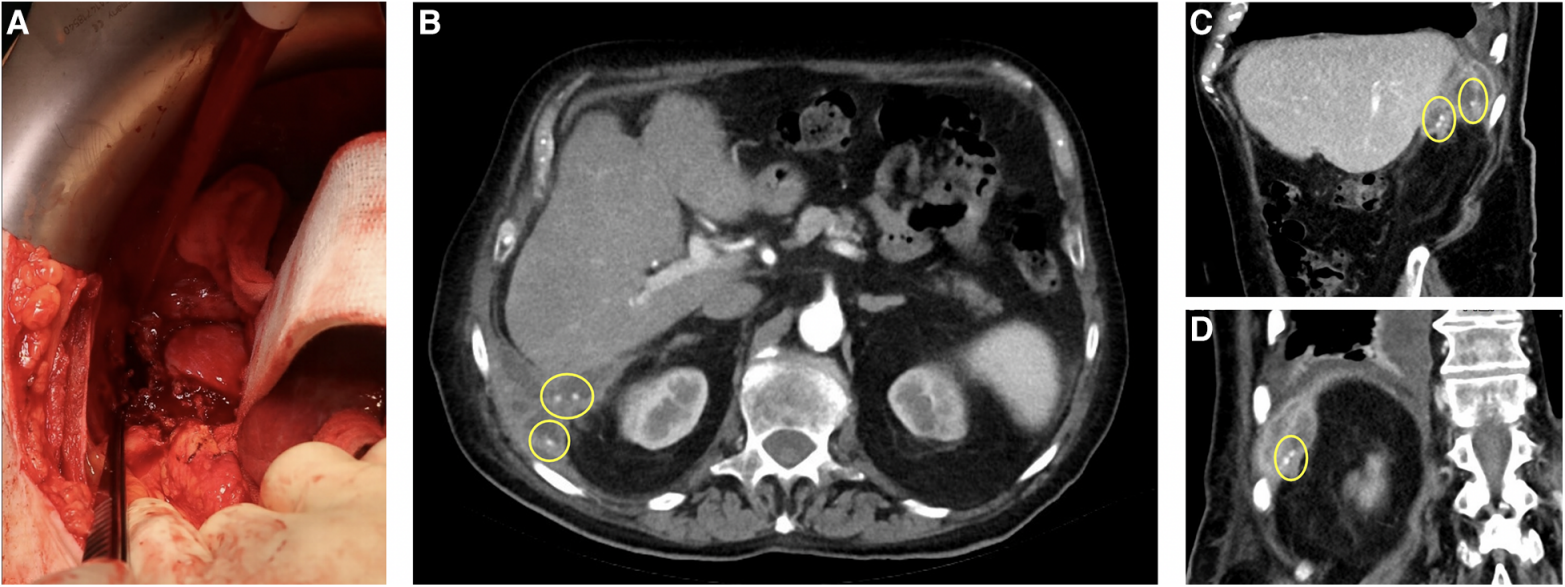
Computed tomography (CT) scan and intraoperative shot of the subhepatic abscess. (A) Macroscopic intraoperative image of the subhepatic abscess. (B,C) Subhepatic abscess in transverse and sagittal sections in CT scan, the lost gallstones are marked. (D) Subhepatic abscess in coronal section in CT scan, perforated diaphragm, right-sided pleural effusion, the lost gallstones are marked.
